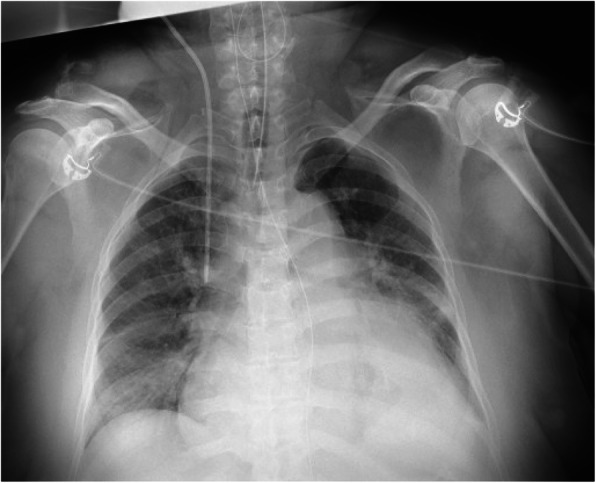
Chest radiography. Cardiomegaly and bilateral costophrenic angle blunting are noted
